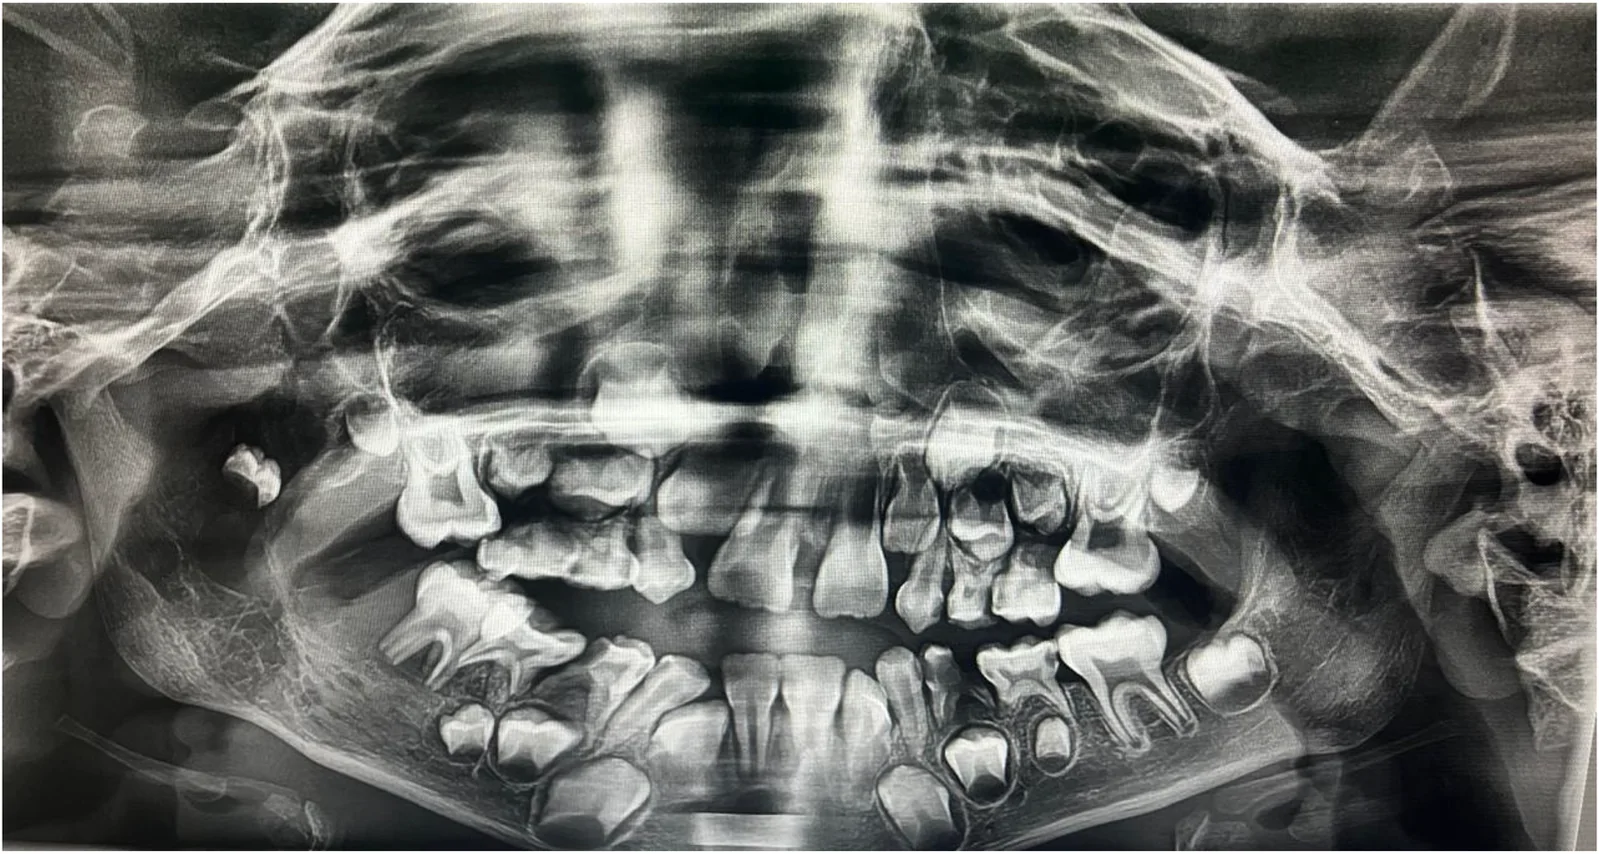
Panoramic radiograph showing: Non-circumscribed radiolucent image involving the body and ascending ramus of the mandible on the right side. 47: Delayed maturation and displacement of the germ in the axis of the ascending ramus on the right side. 46: Arrested root development and interradicular lysis. Tooth buds of 45 and 35: Size abnormality.
